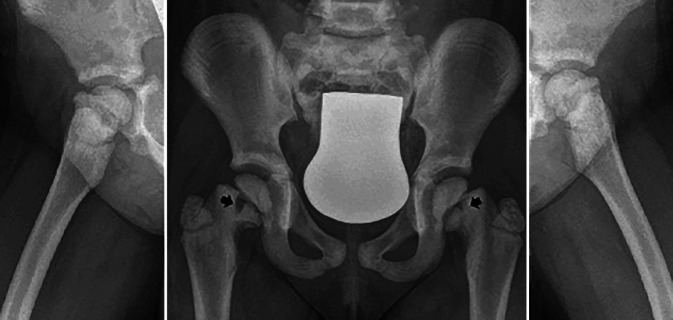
Preoperative anteroposterior view of the pelvis and Lowenstein radiograph showing bilateral femoral neck fractures (Black arrows) with grade I slippage of the growth plates
